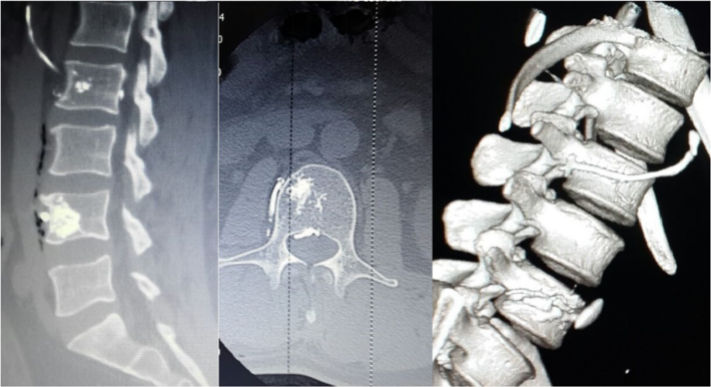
A computed tomography scan showed the characteristic appearance of cement leakage at the level of augmented vertebrae in the perivertebral venous system, segmental vein, epidural space, and soft tissue
